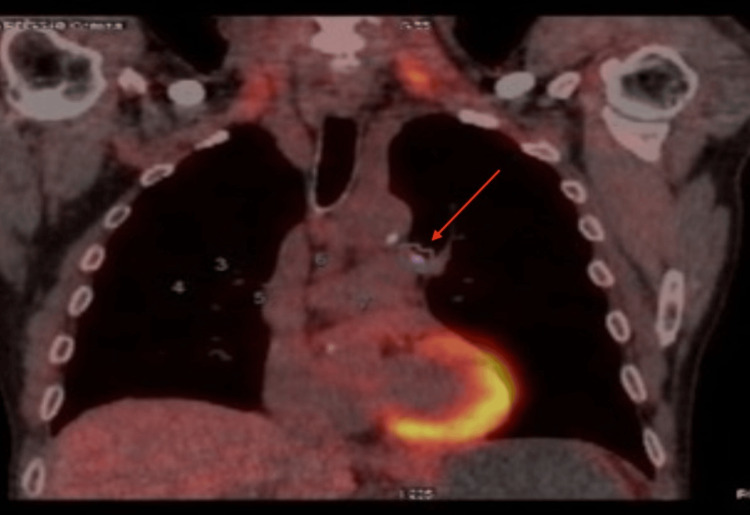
Positron emission tomography-computed tomography scan showing an inflammatory mediastinal adenopathy.
